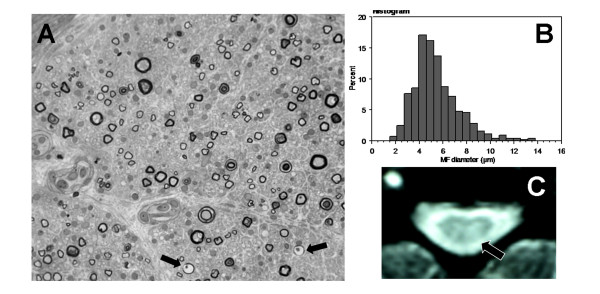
Fiber loss mainly involved large fibers with a few degenerating axons (arrows) and no regenerating cluster as seen in the photograph of the superficial radial nerve biopsy (semithin section × 40) in (A) and the histogram in (B), (C), Abnormal hyperintensity in the left posterior column on the cervical spinal cord T2-MRI.
